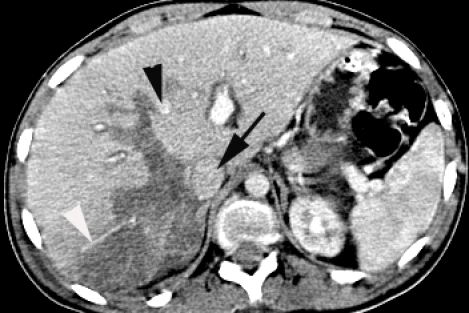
Abdominal CT. Degree Ⅳ liver laceration. White arrowhead – branch of right hepatic vein. Black arrow  – inferior vena cava. Black arrowhead – middle hepatic vein
